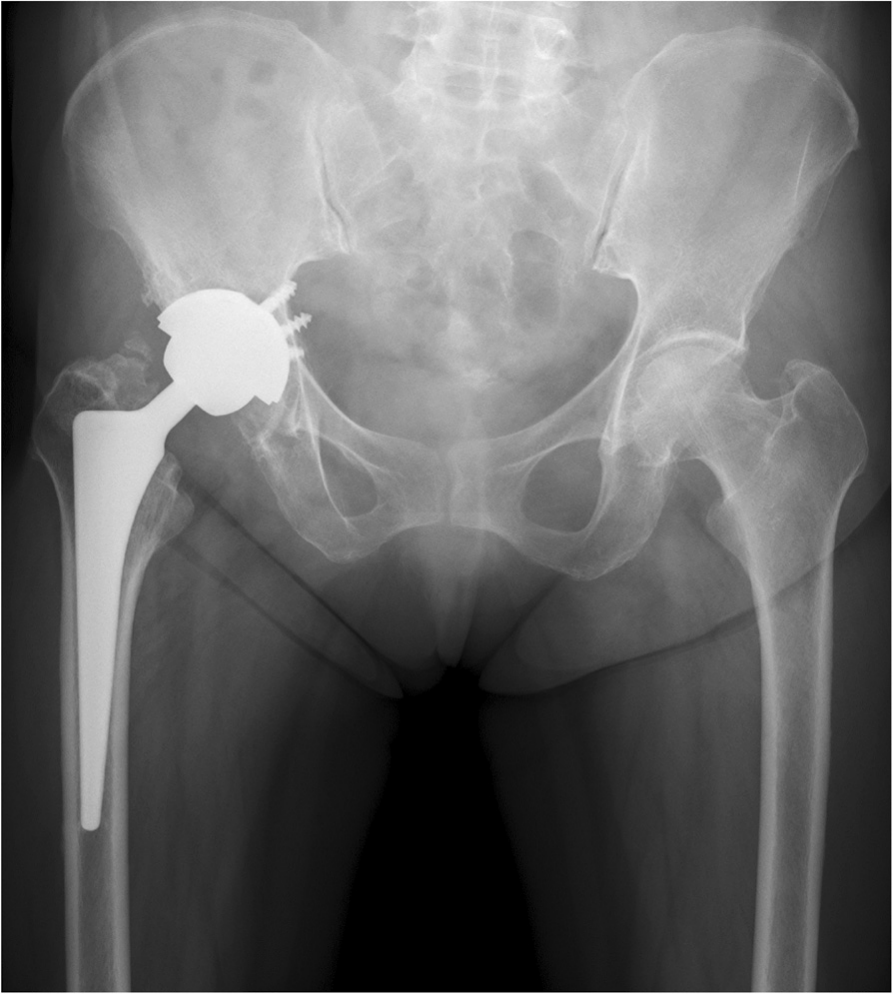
Radiograph taken at the 3-year follow-up of total hip arthroplasty (8 months before the fracture) showing no abnormal findings.
